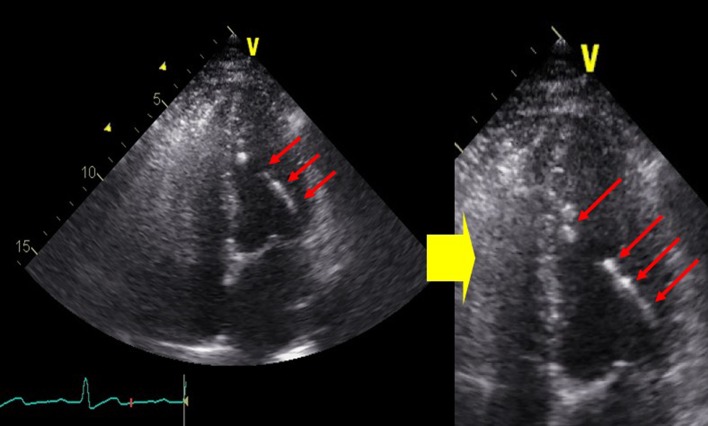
Two dimensional transthoracic echocardiogram showed a spindle-like extensive calcification of anterolateral papillary muscle.
